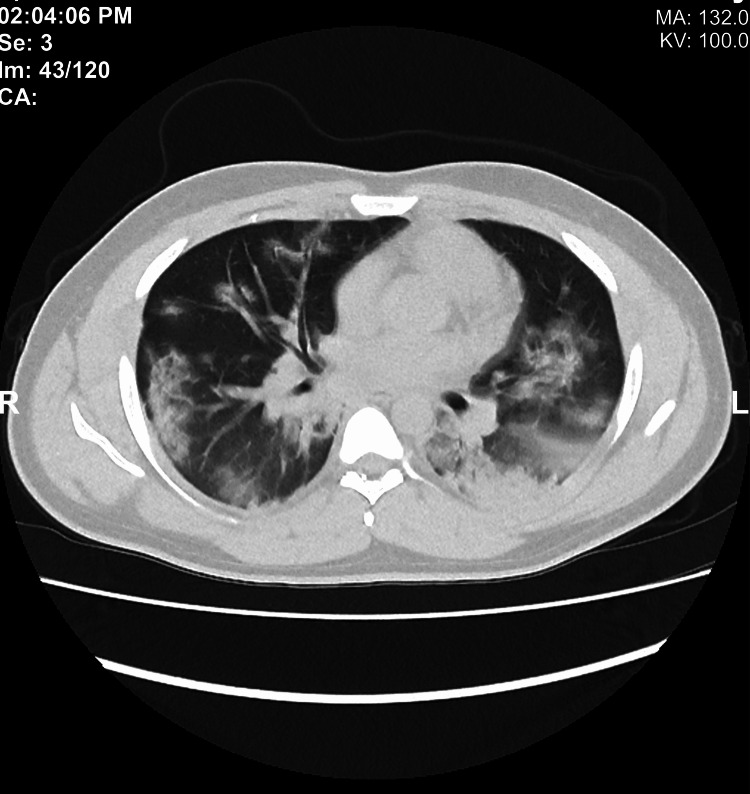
Chest HRCT shows bilateral peripheral ground-glass opacification and consolidation HRCT: high resolution computer tomography
